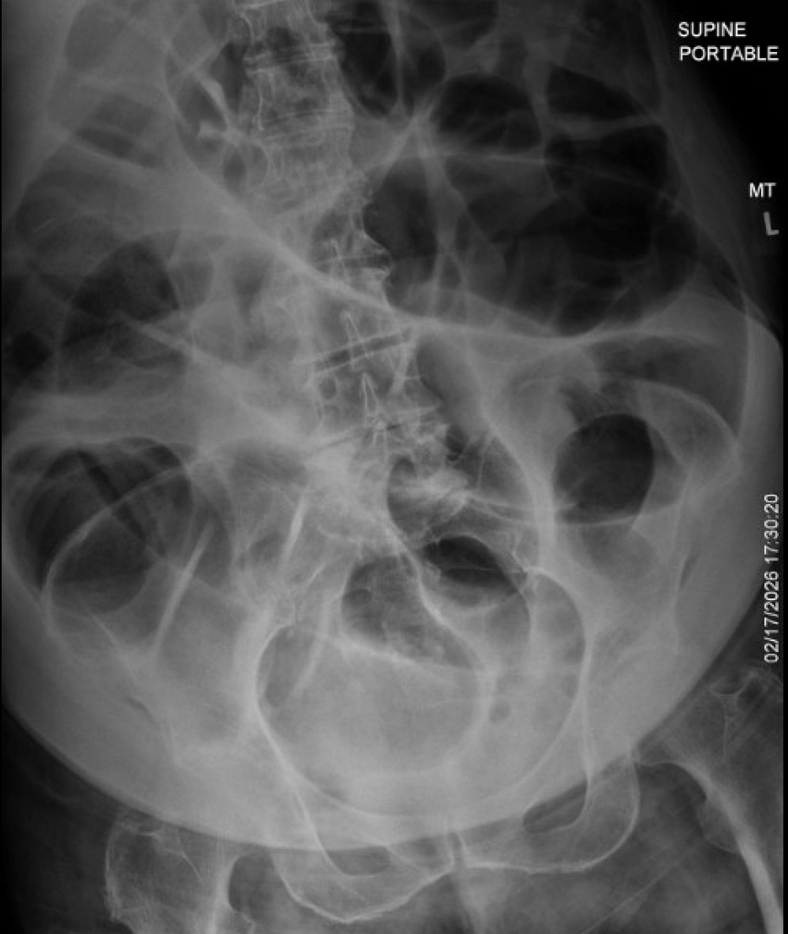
Supine abdominal radiograph obtained at time of emergency department presentation. Dilated gas-filled loops of large bowel, measuring up to 10.8 centimeters.
